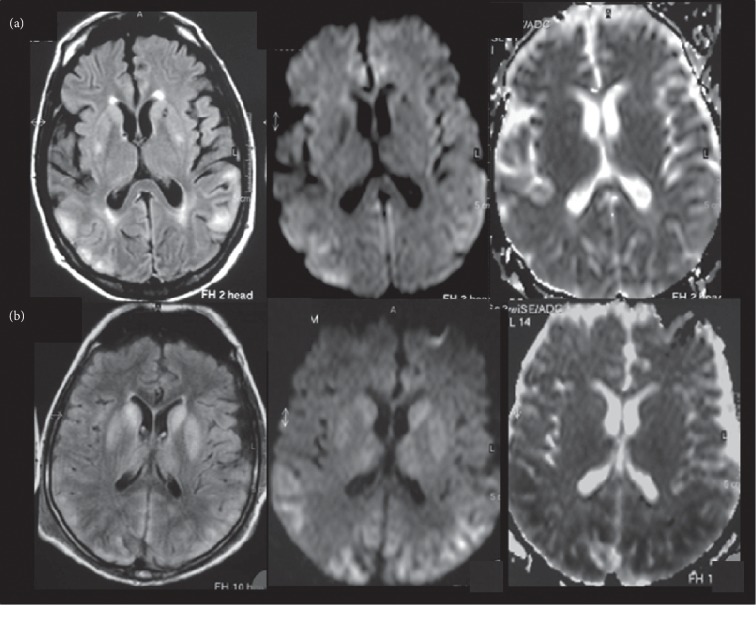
An MRI performed three days after clinical onset showed (a) T2-FLAIR and DWI bilateral and symmetrical hyperintense signal, involving the temporo-parieto-occipital cortex with normal ADC values and (b) T2-FLAIR and DWI which show the similar alterations involving the temporo-parieto-occipital cortex, with decreased ADC values; in this patient, hyperintensity in the T2-FLAIR and DWI is also evident, involving the basal ganglia and thalami without alterations on ADC maps.
